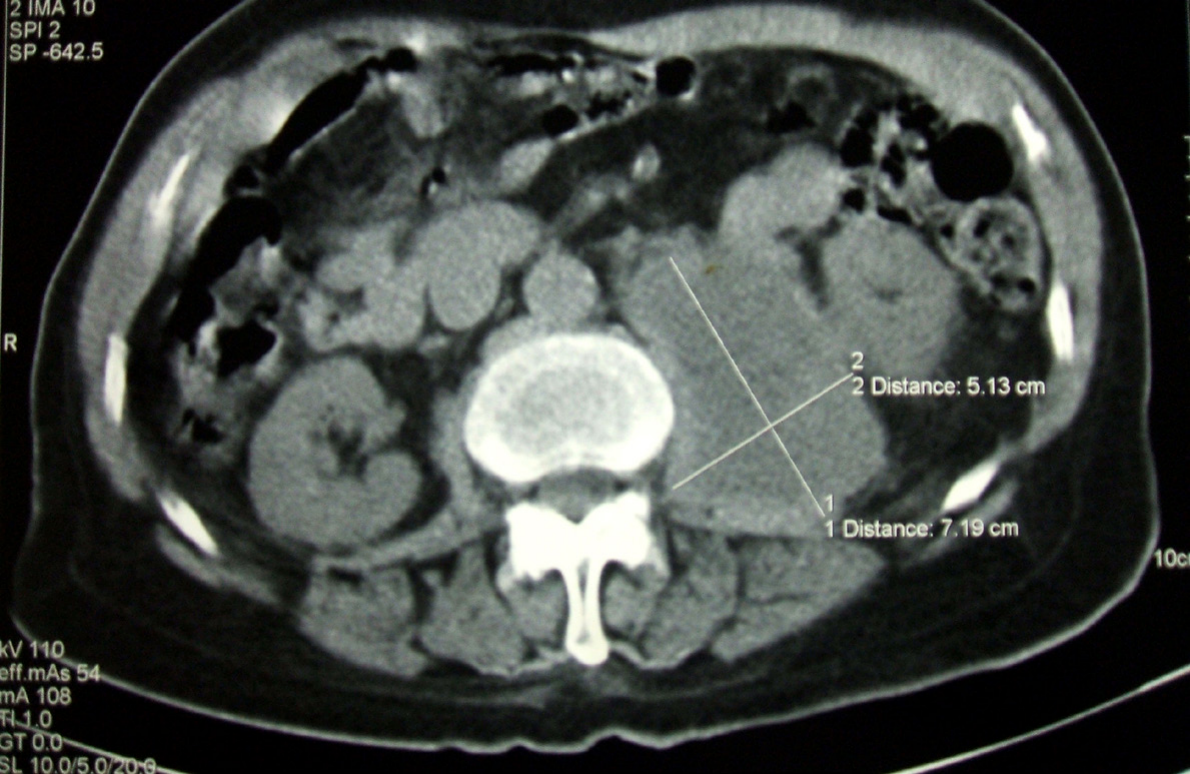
Contrôle tomodensitométrique après ponction scanner guidée CT scan control following guided puncture
